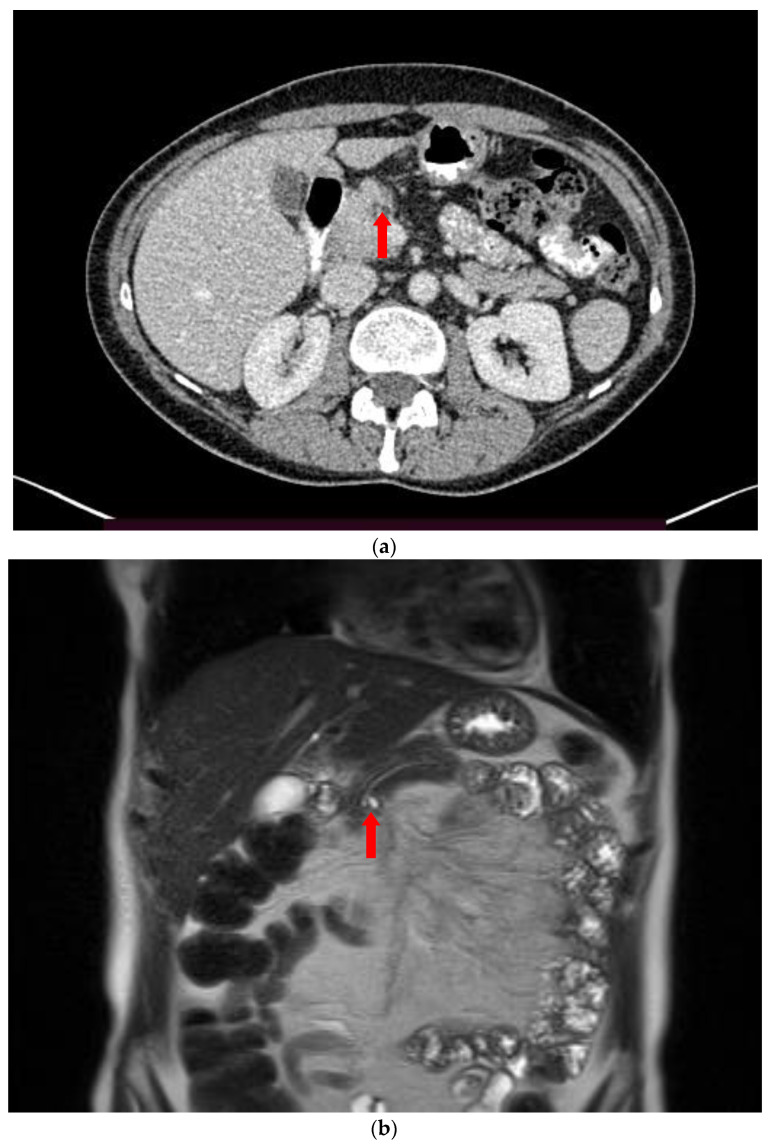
Contrast-enhanced CT image (a) and coronal T2-w MR image (b), show a small branch duct IPMN (arrow) communicating with the main duct. The IPMN is more easily seen on the MR image.
